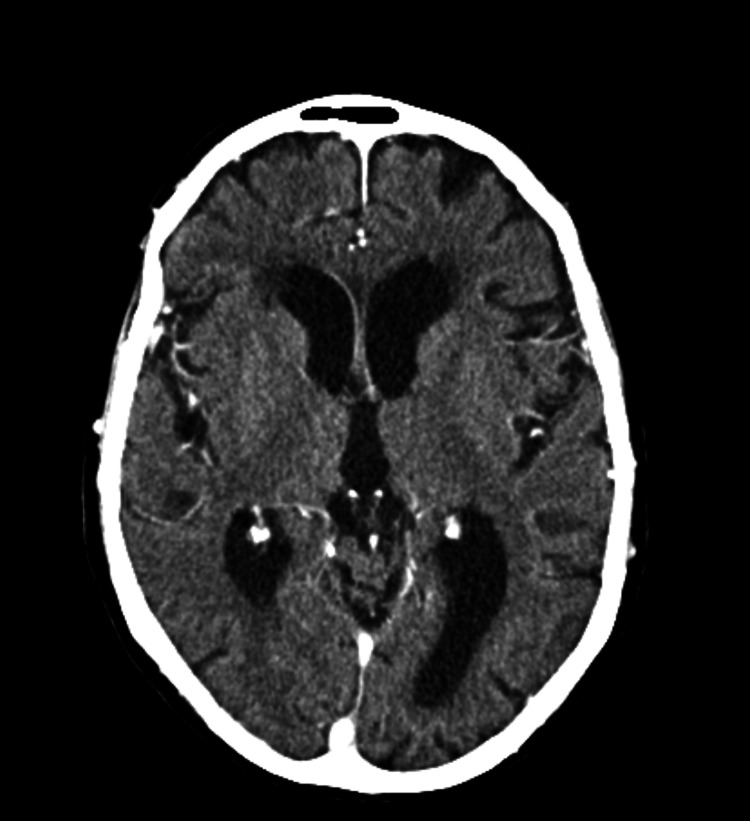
Control computed tomography of the cerebral vessels 30 days after the first event.
